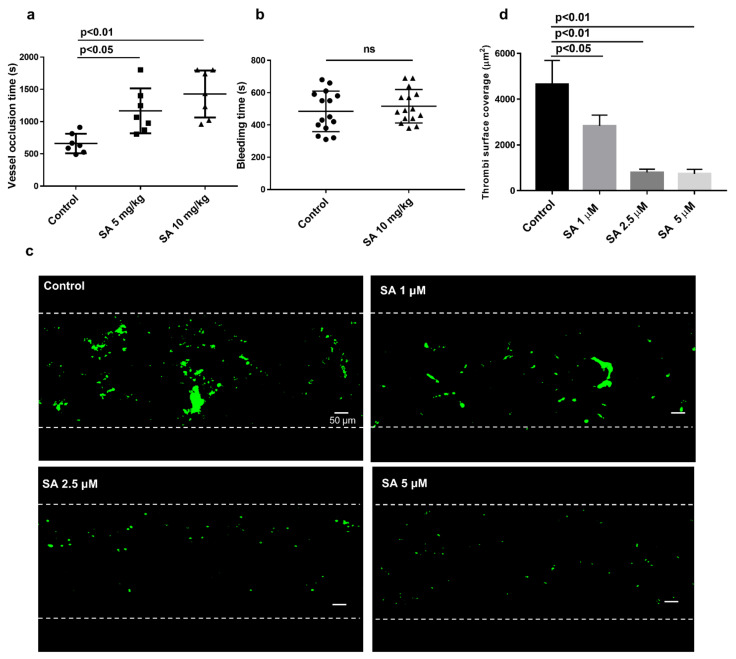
Sanguinarine inhibits the formation of thrombus in vivo and in vitro without prolonging bleeding time. (a) Summary data of ferric chloride (FeCl_3_)-induced thrombus formation. Thrombus formation in vivo was assessed by occlusion time(s) after FeCl_3_ -induced injury of the carotid artery, which was measured with a doppler flow probe. The occlusion time of the sanguinarine-treated (5 mg/kg or 10 mg/kg, i.g.) were compared with the control (saline + 5% DMSO i.g.) group. The results were expressed as mean ± SEM (*n* = 7) and were analyzed using one-way ANOVA. (b) The effect of sanguinarine on bleeding time. Results are shown as mean ± SEM (*n* = 15), and data were analyzed using an unpaired t test. (c) Sanguinarine reduced thrombosis in vitro. Washed human platelets were labeled with CellTrace Calcein Green and then incubated with DMSO (control) or sanguinarine (1, 2.5 and 5 μM) for 10 min. The mixture of labeled platelets and washed red blood cells (with a hematocrit of about 40%) and CaCl_2_/MgCl_2_ (75 mM/37.5 mM) was perfused through Bioflux plates at a wall shear rate of 2000 s^−1^ for 5 min. Representative images of surface coverage were revealed. (d) Summary data of area covered by platelets at the 5 min time point were studied and measured (scale bars, 50 μM). Results and data were collected from at least five separate independent experiments, analyzed using one-way ANOVA analysis and presented as mean ± SEM (*n* = 5).
